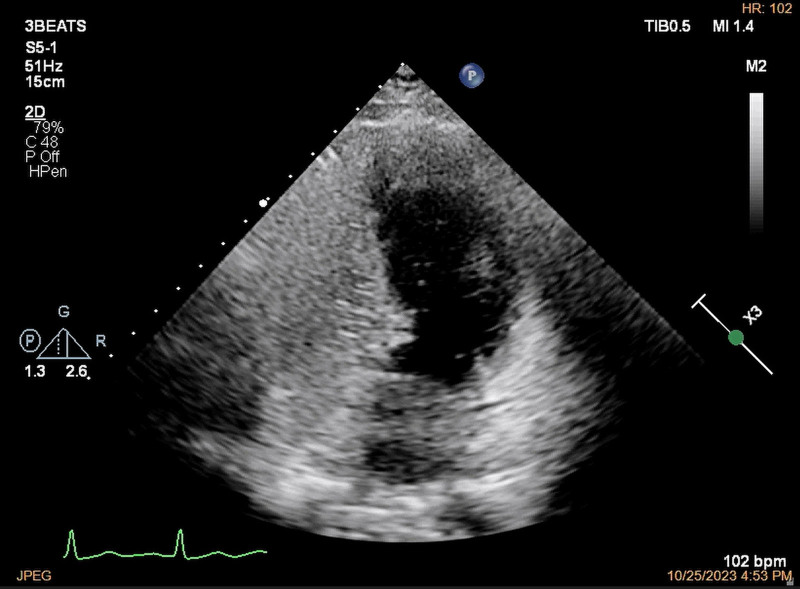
The apical 4-chamber view showed that <5 microbubbles were found at the left-sided heart chambers during 10 cardiac cycles after the effusion of the right-sided heart chambers by agitated saline contrast transthoracic echocardiography through left median cubital vein.
